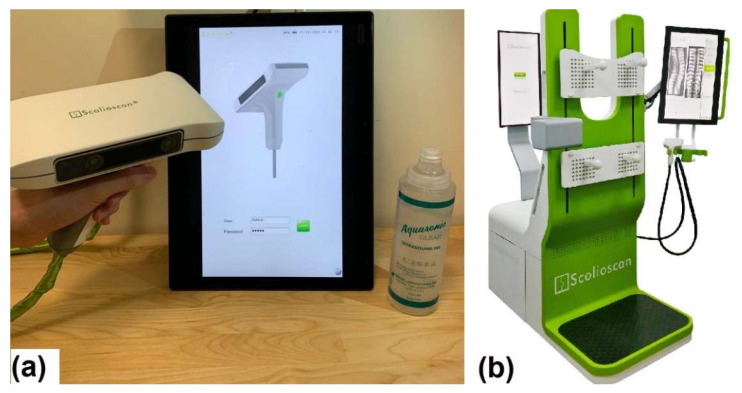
Pictures showing (a) portable 3D ultrasound imaging system Scolioscan Air and (b) conventional 3D ultrasound imaging system Scolioscan [53].
